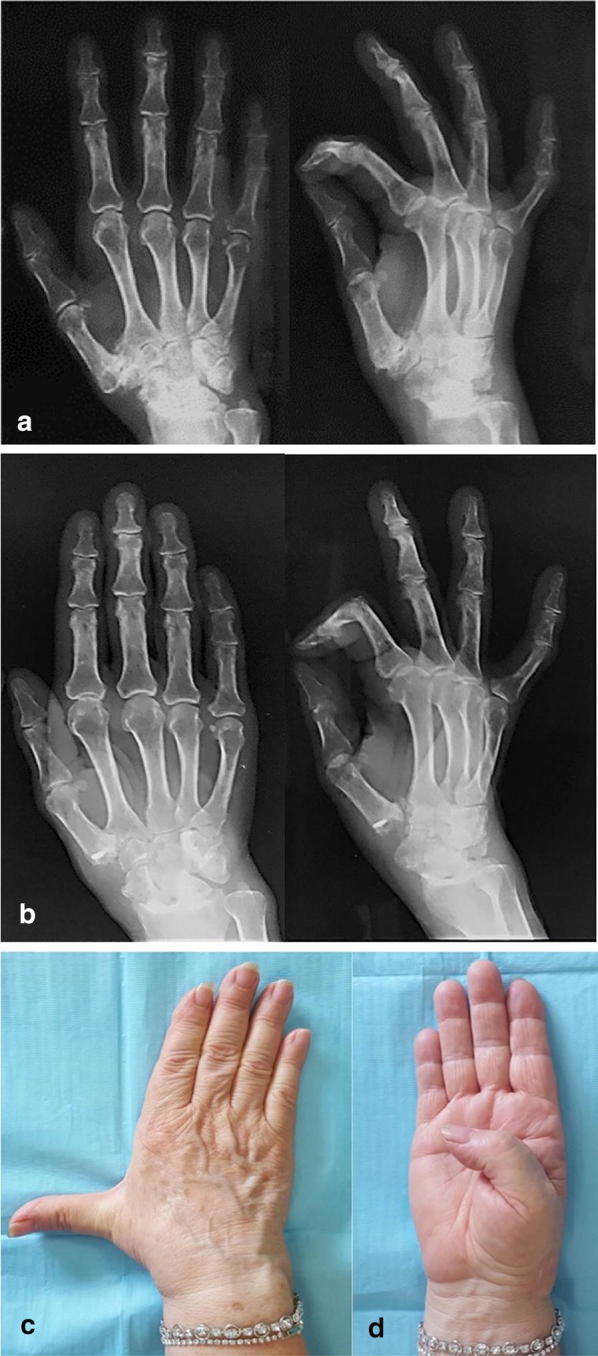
a Preoperative radiographs of the right hand showing severe trapezio-metacarpal osteoarthritis in a 57-year-old woman, complicated by pain and disability of the thumb. b At follow-up, 9 years after surgery, X-rays did not show proximal migration of the thumb metacarpal or signs of impingement on the scaphoid. c, d Clinical evaluation showed excellent abduction and joint opposition of the thumb. Metacarpophalangeal hyperextension was evident, as is often observed as a consequence of this surgical procedure. In spite of the hyperextension of the thumb, the patient reported no pain or significant limitations in her daily activities
